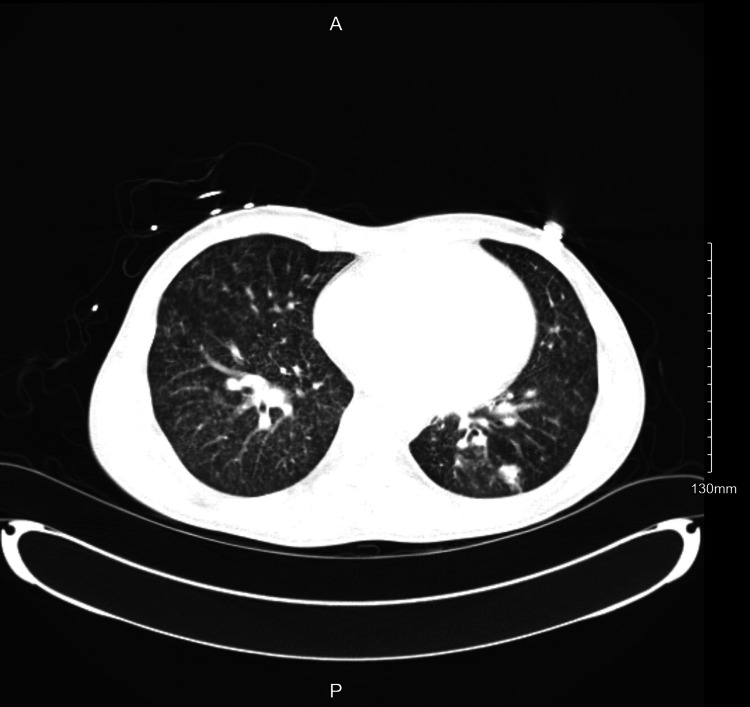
CT chest showing innumerable tiny pulmonary miliary nodules, moderate left lower lobe atelectasis and consolidation, moderate mediastinal adenopathy, and mild retroperitoneal adenopathy.
